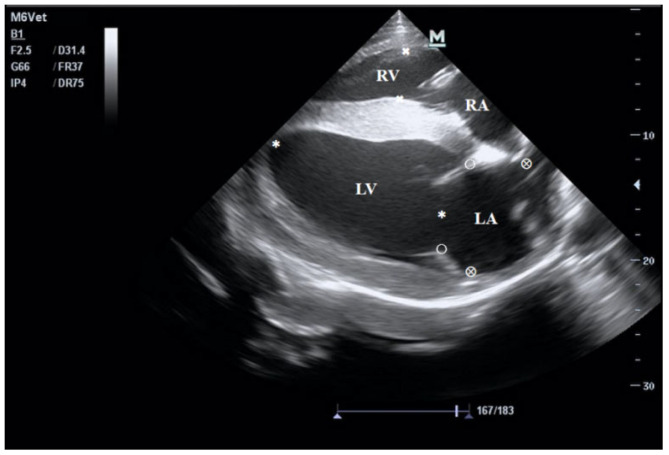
B-mode echocardiogram of the right parasternal long-axis four-chamber view. LV: left ventricular; RV: right ventricular; RA: right atrium; LA: left atrial.
